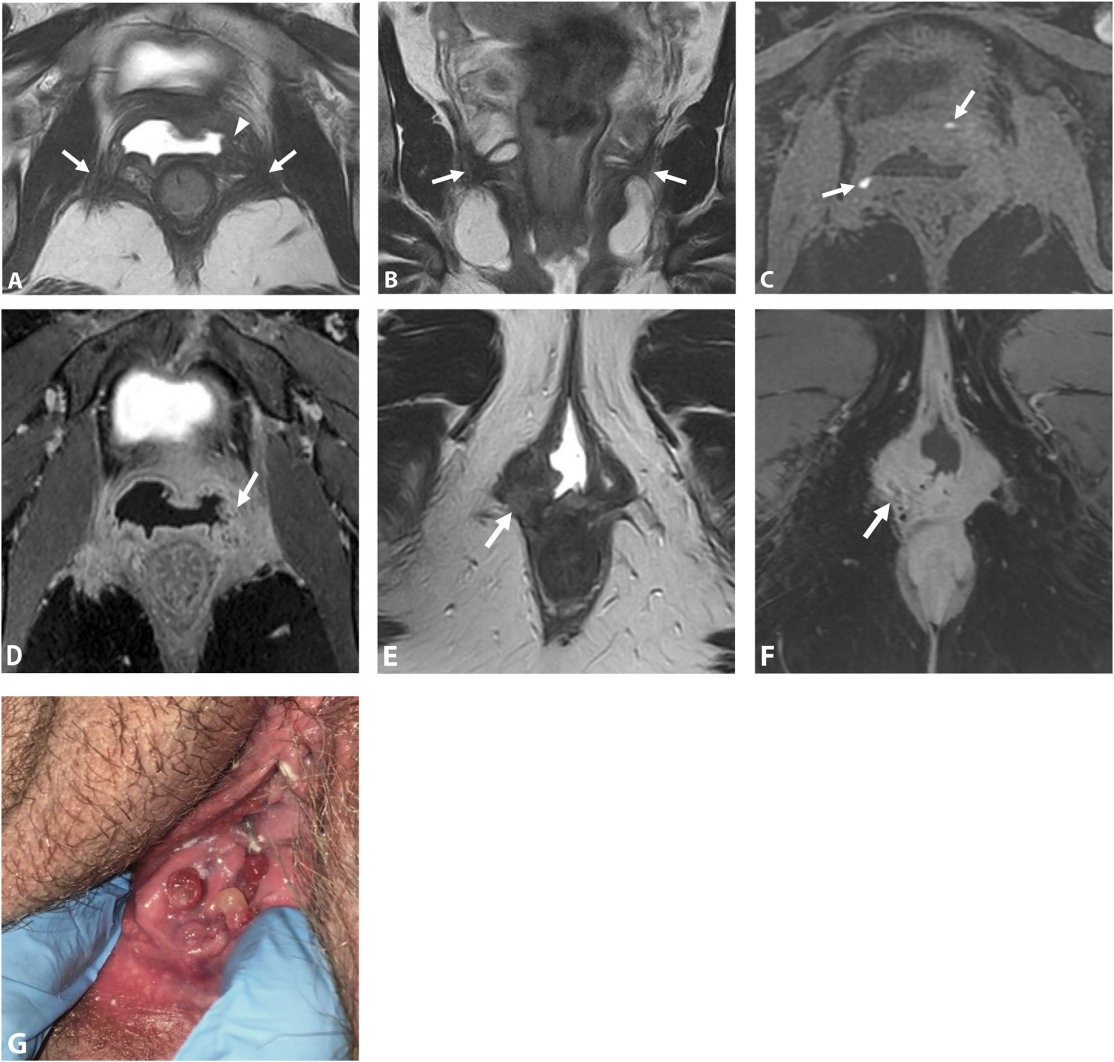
DE in a 34-year-old woman with pudendalgia after vaginal delivery with forceps extraction and bilateral mediolateral episiotomy. A Axial and (B) coronal T2W MR images show a bilateral fibrotic infiltration (arrows) of the levator ani muscle at the level of the distal course of bilateral pudendal nerves, as well as infiltration of the left part of the vagina (A, arrowhead). C Axial fat-suppressed T1W MR image reveals some T1-hyperintense endometriotic hemorrhagic microcysts (arrows). D Axial T1W post-contrast MR image shows a better delineation of the left vaginal (sub) mucosal involvement (arrow). E Axial T2W and (F) axial fat-suppressed T1W post-contrast MR images show a right vulvar lesion (arrows) correlating with clinical photographs (G). The patient was treated medically with hormone therapy
